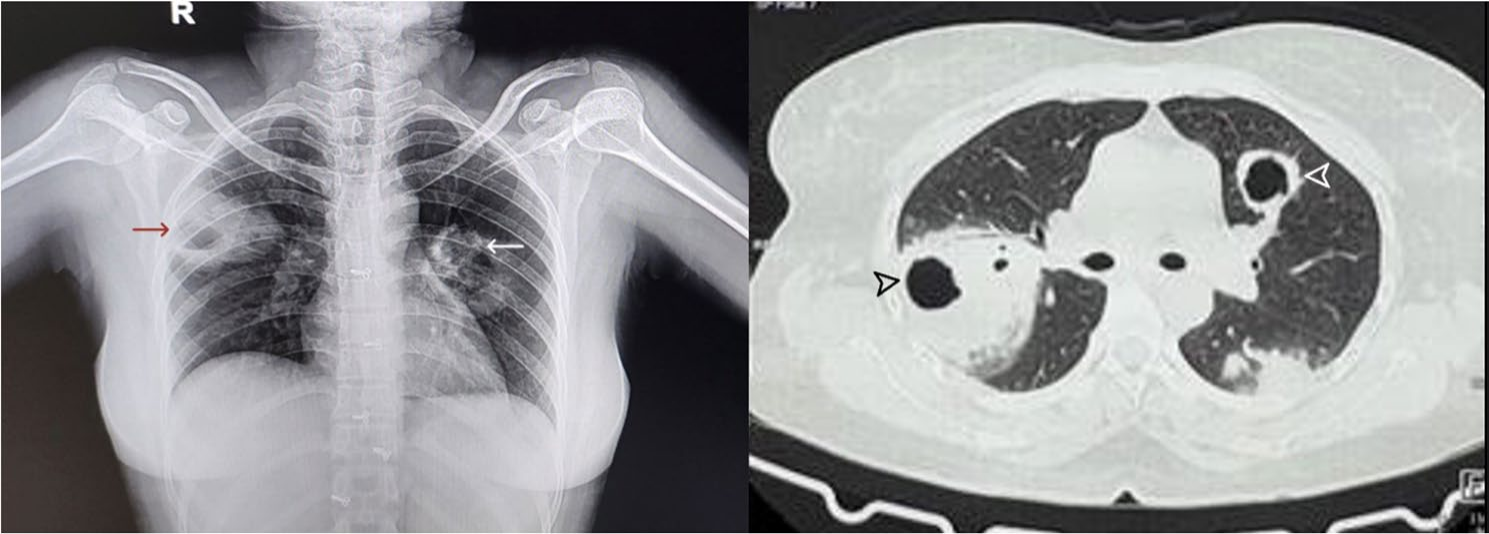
Chest x-ray and HRCT chest showing multiple large cavitating nodules in the posterior segment of the right upper lobe, superior lingular segment of left upper lobe, and superior segment of left lower lobe, with adjacent ground glass attenuation and soft tissue density nodules
